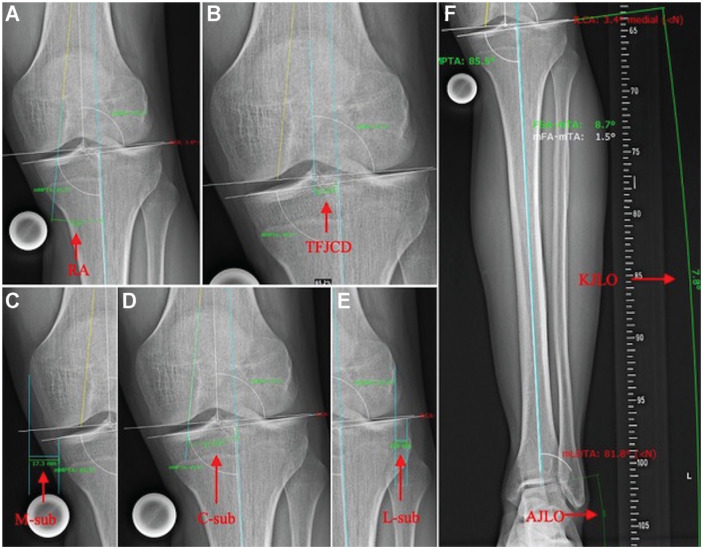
Radiographic measurements of lower limb alignment: (A) RA, the minor angle between the distal femoral and proximal tibial axes; (B) TFJCD, the distance between the center of the tibial JL and the center of the femoral JL on the tibial JL; (C) M-sub, the distance between a strictly vertical line through the most medial point of the medial femoral condyle and a vertical line through the most medial point of the medial tibial plateau; (D) C-sub, the distance between the intersection of the femoral anatomic axis and the tibial anatomic axis at the tibial JL; (E) L-sub, the distance between a strictly vertical line through the most lateral point of the lateral femoral condyle and a vertical line through the most lateral point of the lateral tibial plateau; and (F) KJLO, the angle between the tibial JL and a horizontal line parallel to the floor; and AJLO, the angle between the talar JL and a horizontal line parallel to the floor. AJLO, ankle joint line obliquity; C-sub, central subluxation; JL, joint line; KJLO, knee joint line obliquity; L-sub, lateral subluxation; M-sub, medial subluxation; RA, rotation angle; TFJCD, tibiofemoral joint line center distance.
